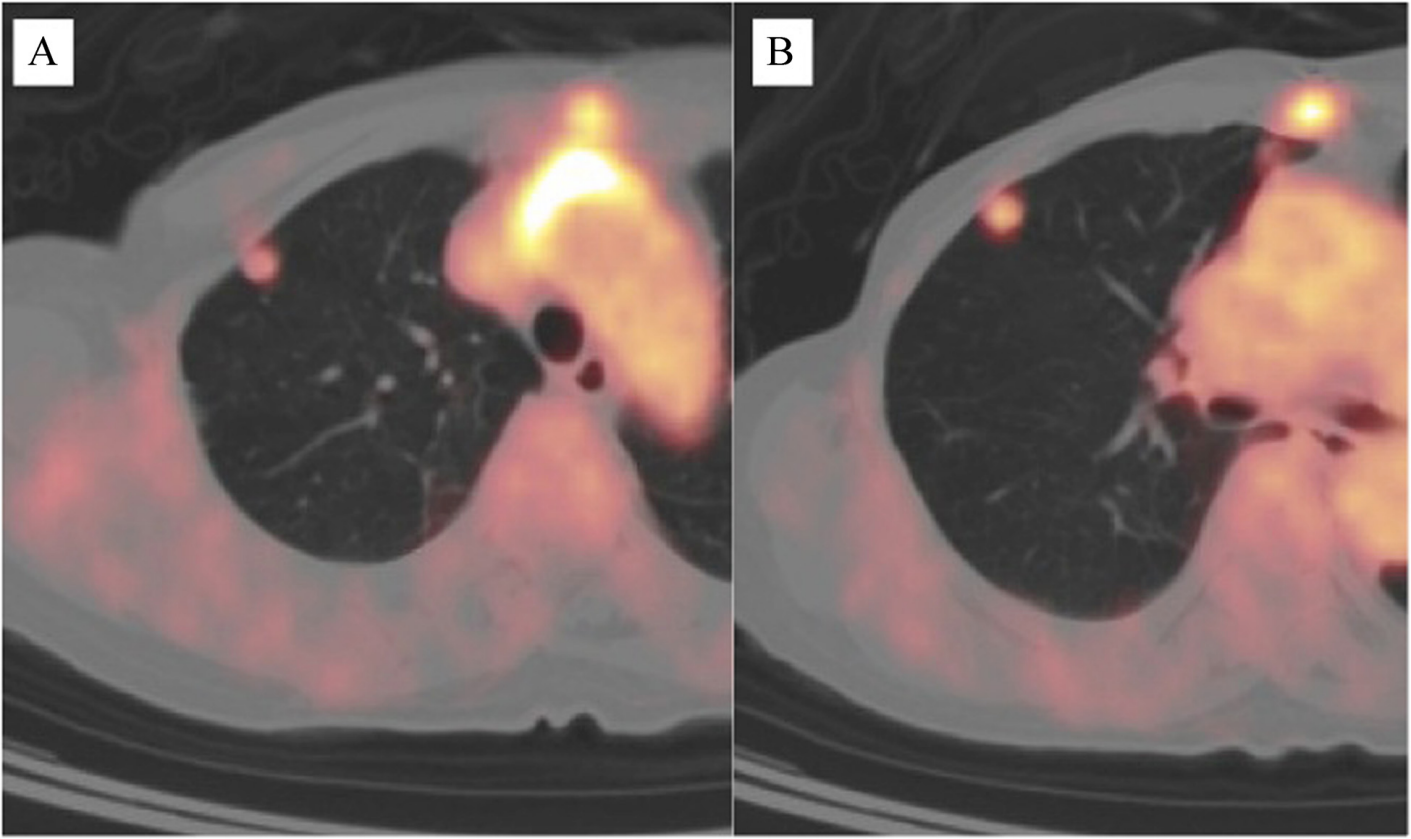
FDG-PET showed abnormal accumulation in the right S1 tumor (SUV max 2.35) (a) and in the right S3 tumor (SUV max 3.45) (b)
